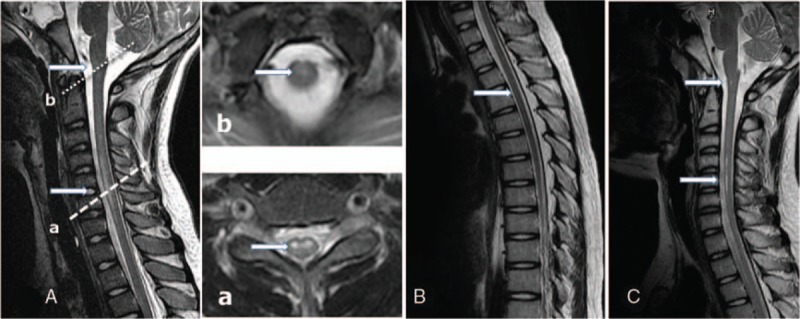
Serial MR images of T2-weighted images. A, At admission, longitudinally extensive cord lesions mainly involving the gray matter with hyperintensity and swelling from thoracic to cervical spinal cord (T1-C3) and conus medullaris (a, b). B, Two months later after the onset, diffused increased signal intensity on T2WI from cervical spinal cord to conus medullaris without enlargement or atrophy. C, Five months later after the onset, small dot and linear-like cavities are seen in the cervical spine and cervicomedullary junction. MR = magnetic resonance.
